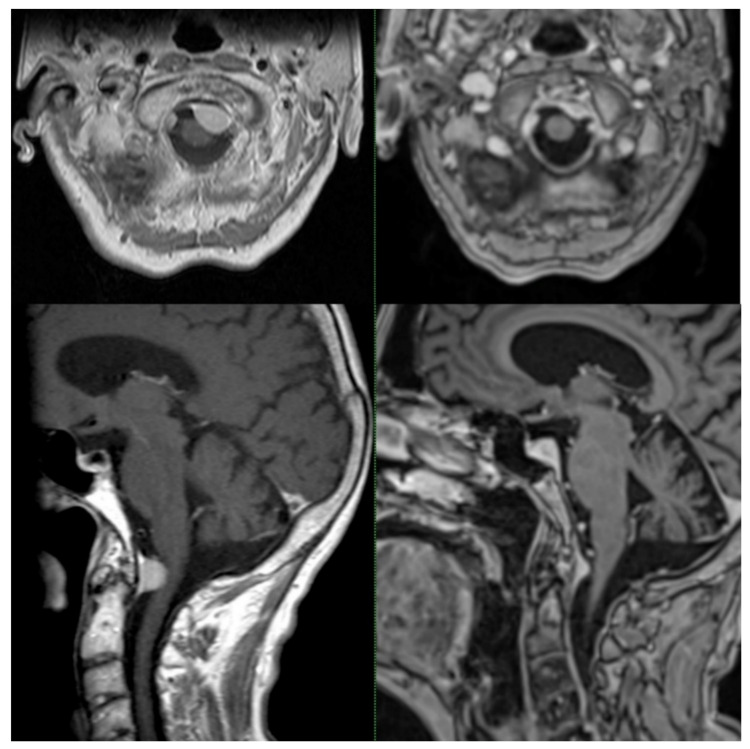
Axial and sagittal pretreatment (left top and bottom) and posttreatment contrast-enhanced MRI (right top and bottom). Incidental finding of a FMM in a 79-year-old man without any neurological findings. To avoid future tumor-associated complications and with respect to the age and comorbidities of the patient, SRS of the FMM was conducted in one session with 13.5 Gy prescribed to the 70% isodose line. After a follow-up of 67 months, the tumor volume decreased from 2.1 to 0.6 cc. No new neurological deficits developed after treatment delivery.
